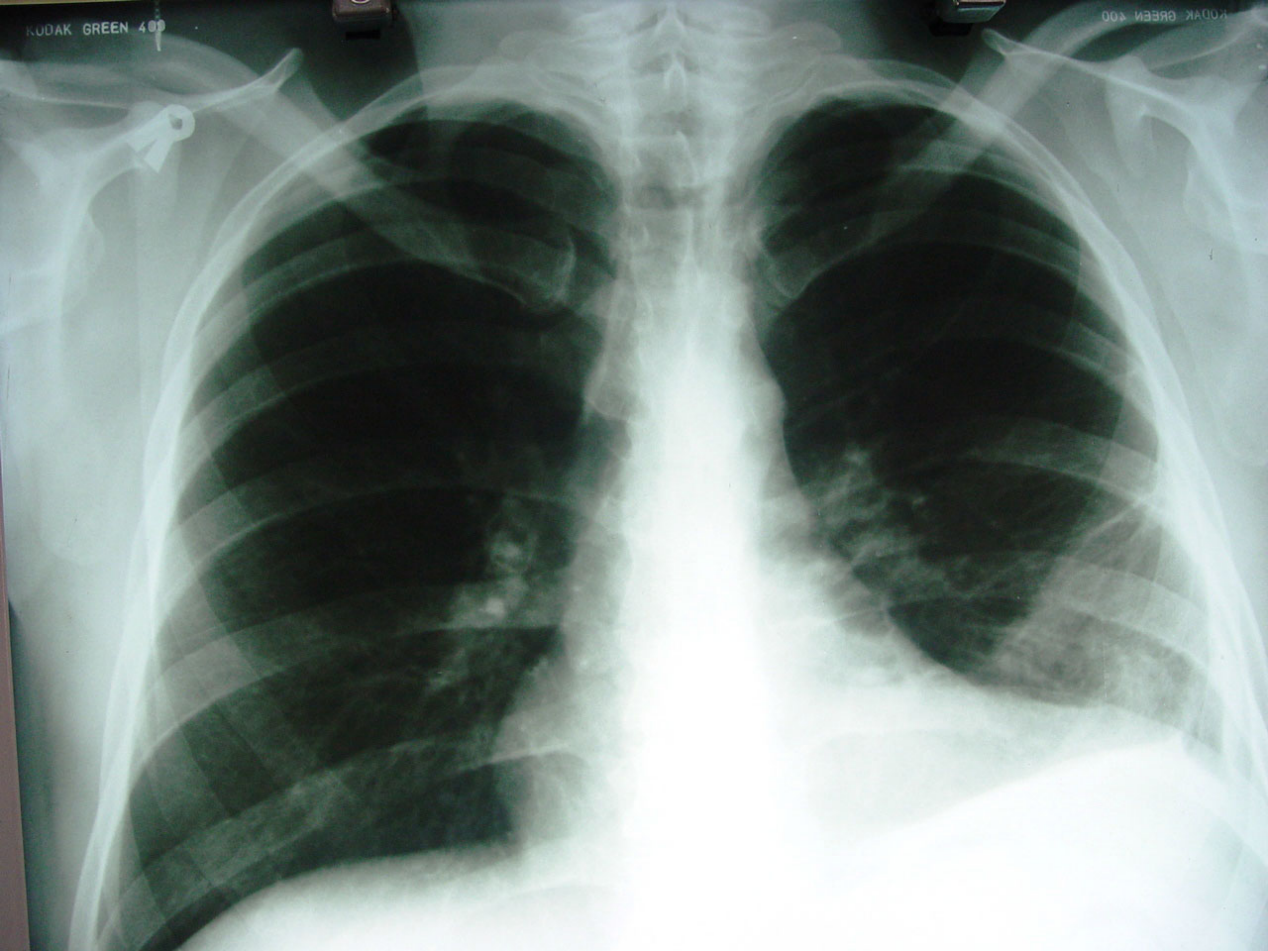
Chest X-ray showing consolidation in the lower lobe of the left lung.
